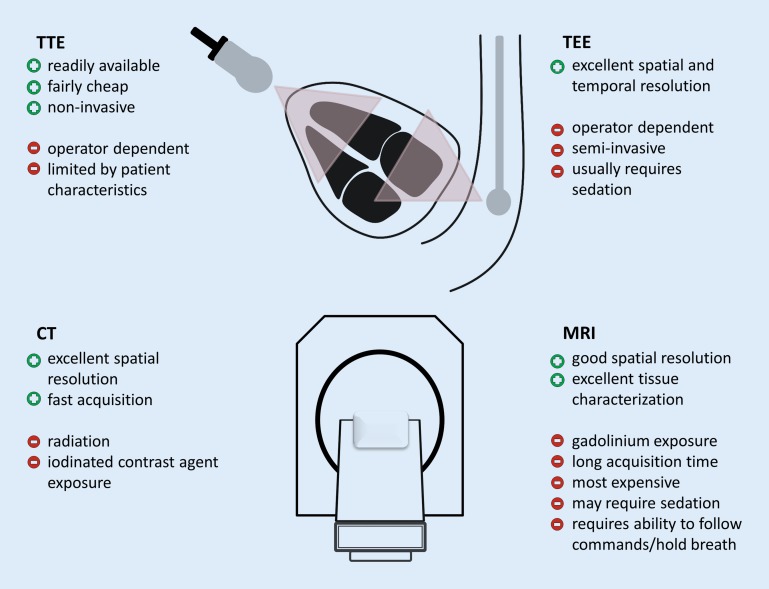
Advantages (+) and disadvantages (−) of different cardiac imaging methods. General benefits and limitations of transthoracic echocardiography (*TTE*), transesophageal echocardiography (*TEE*), cardiac computed tomography (*CT*), and cardiac magnetic resonance imaging (*MRI*) are shown
